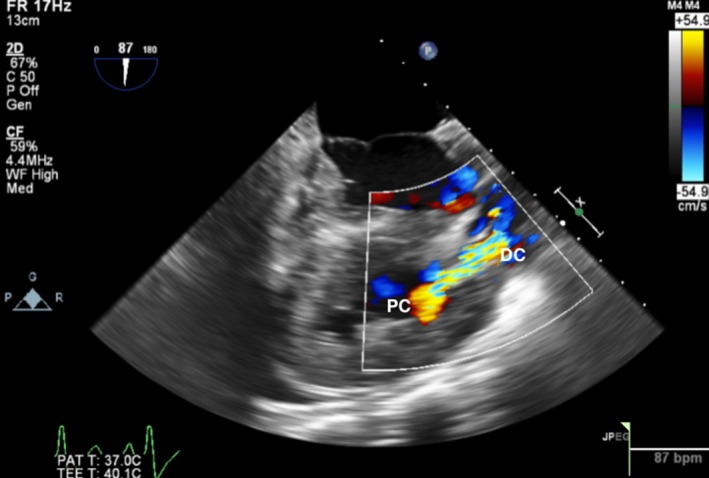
Transesophageal echocardiogram with Doppler signal showing flow acceleration across the proximal chamber (PC) and distal chamber (DC)
